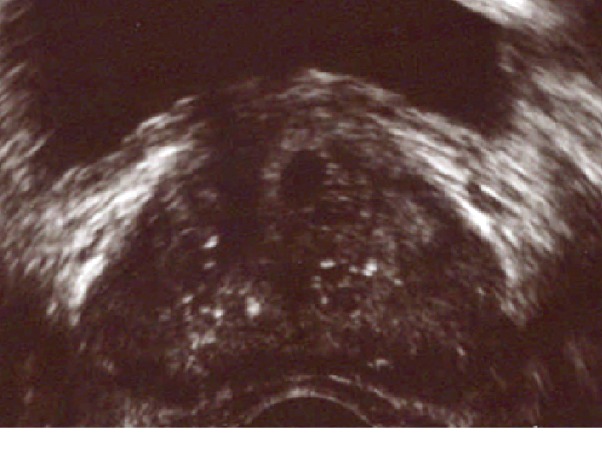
Image obtained using transrectal ultrasound at the second biopsy showing an enlarged prostate (axial view). The estimated prostate volume was 49 cm^3^. Magnetic resonance imaging was not performed because the patient had undergone femoral head replacement.
